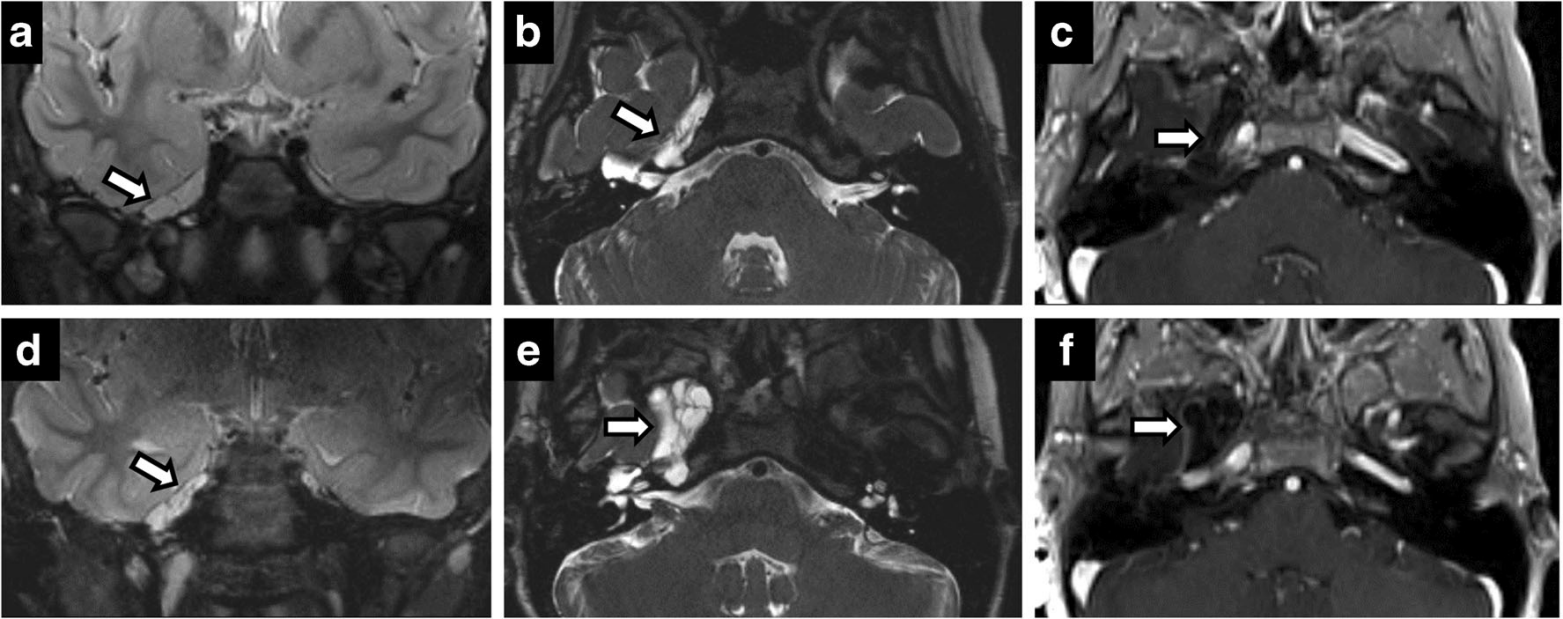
Petrous apex cephalocele. Coronal (a, d) and axial (b, e) T2-weighted images of a cystic petrous apex lesion that communicates with the posterolateral portion of Meckel’s cave. No abnormal enhancement on axial post-contrast images (c, f)
